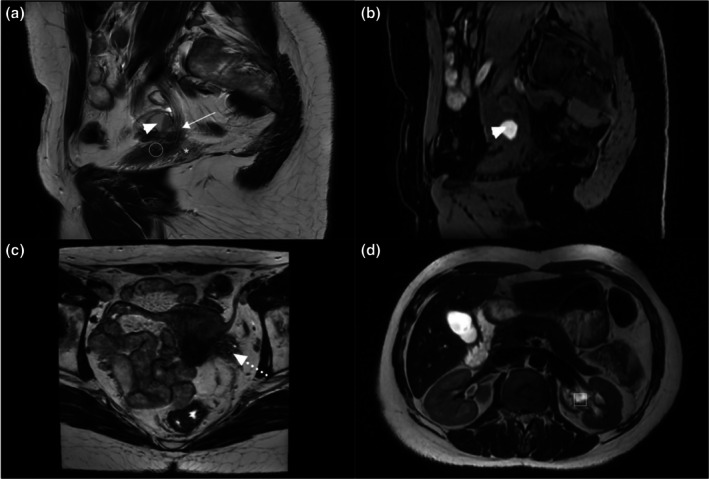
A 32‐year‐old patient with endometriosis involving lateral subperitoneal structures. 3D T2‐weighted magnetic resonance imaging (MRI) sequences, sagittal slices (a) showing ureteral involvement (white arrow) with an intraluminal JJ stent, adjacent parametrial involvement (white star), and bladder involvement (white circle). Notably, an adjacent endometrioma (white arrowhead) with hyperintense signal corresponding to the T1 WATER‐weighted MRI sequences, sagittal slices (b) (white arrowhead). Corresponding involvement in 3D T2‐weighted sequences, axial slices (dotted white arrow) (c), and dilation of the pyelic cavities of the upstream left kidney (white rectangle) (d).
